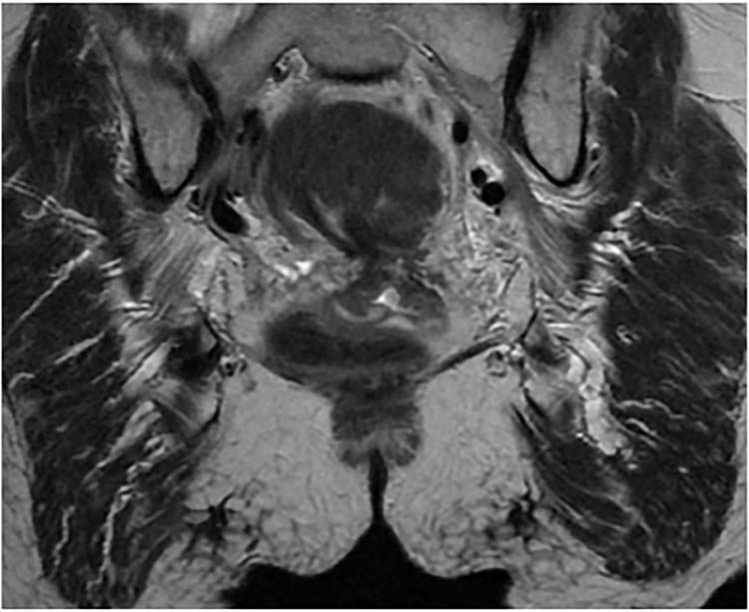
1.5 pelvis MRI, coronal view demonstrates apple core lesion at 5 cm from anal verge staged at T3N0 without involvement of anal sphincter.
